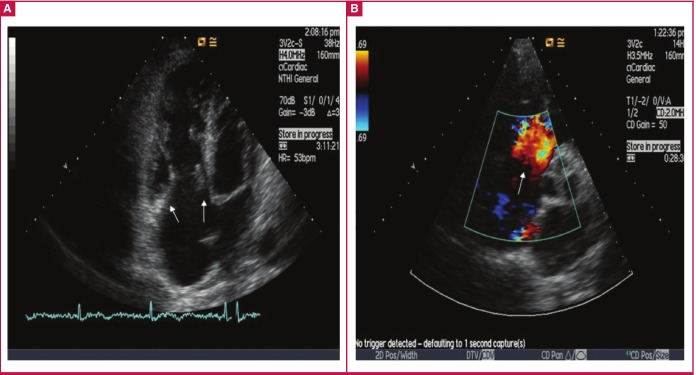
Patient 1. (A) Apical four-chamber view showing tricuspid leaflets that are thickened and retracted (arrows). The right ventricle and atrium are dilated. (B) Colour Doppler with free flow through the tricuspid valve during systole in a parasternal short-axis view at the level of the aortic valve (arrow).
